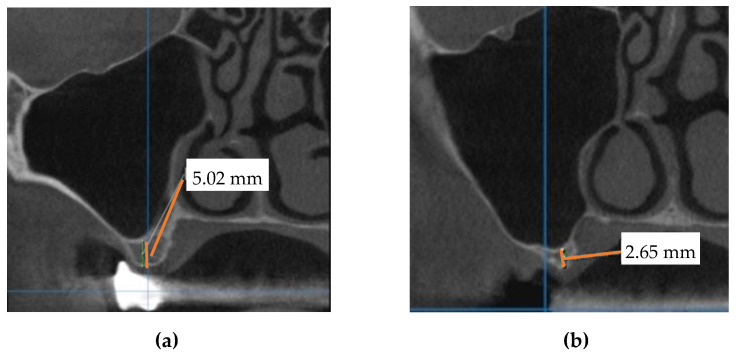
Cross-sectional CBCT views corresponding to: (a) the 16 with a bone height of 5.02 mm; (b) the 17 with a bone height of 2.65 mm and insufficient crestal width.
